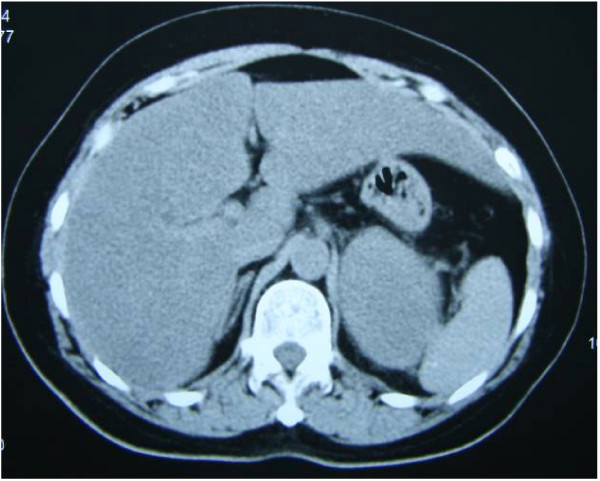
Unenhanced CT scan showing a 5.4 × 7.0 cm, homogenous, low-density (27 HU) mass of the left adrenal.
